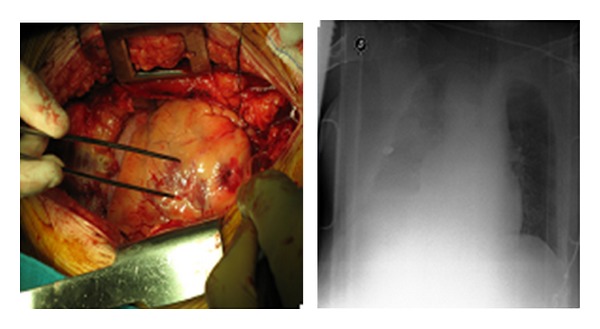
GSW to the right ventricle. Chest X-ray showing a bullet in the right hemithorax and a massive right hemothorax.
